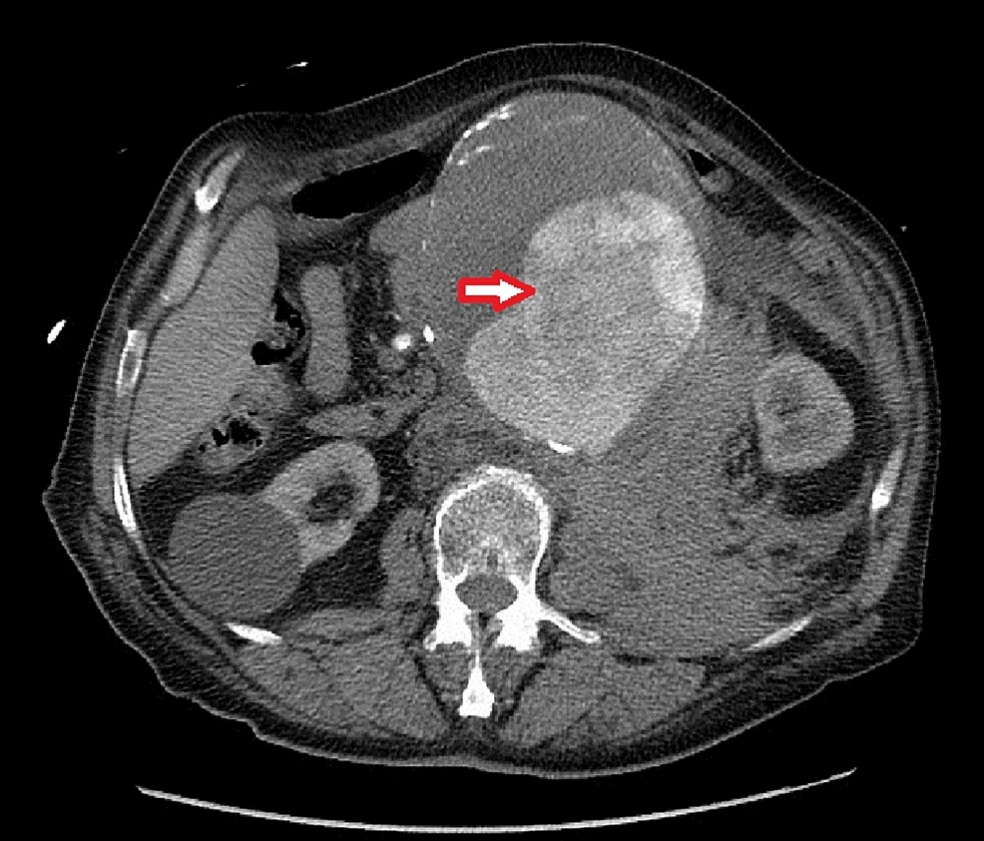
Computed tomography (axial view) showing significant aneurysm in the abdominal aorta with minimal surrounding stranding, suggesting an early or slow rupture. Despite the limited evidence of blood leakage, this condition remains a surgical emergency
